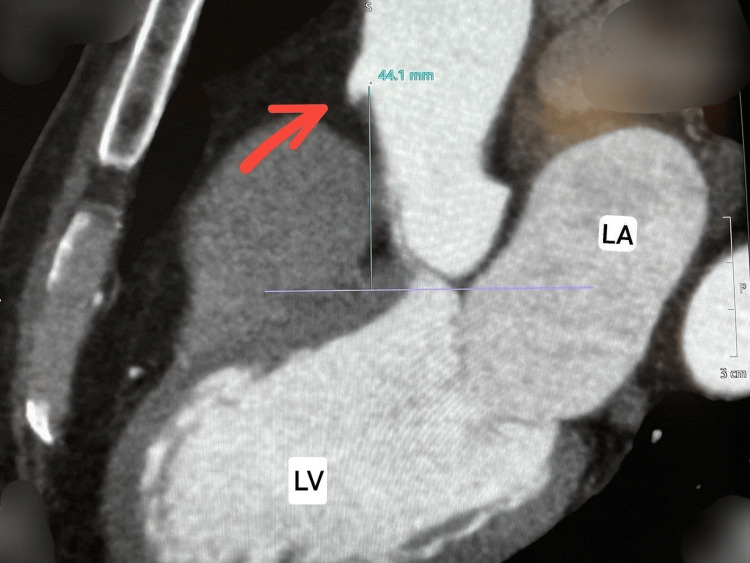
Coronary CT scan image LA: left atrium; LV: left ventricle; RCA: right coronary artery Red arrow: ostial RCA that originates at 44 mm above the aortic cusp
